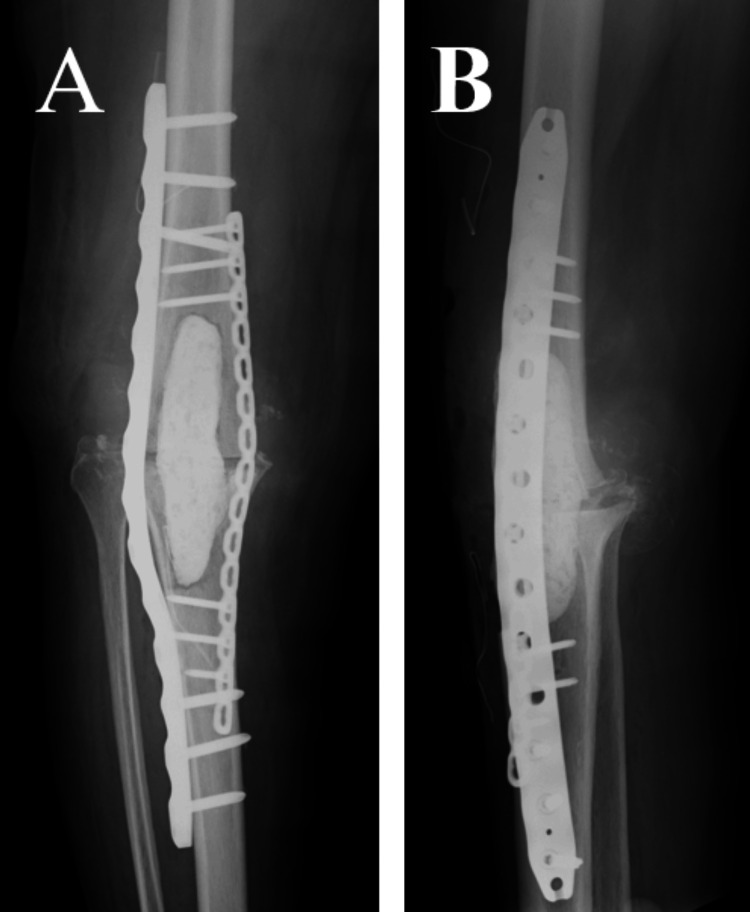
First-stage postoperative X-ray (A) Anteroposterior radiograph of the knee joint. (B) Lateral radiograph of the knee joint.
